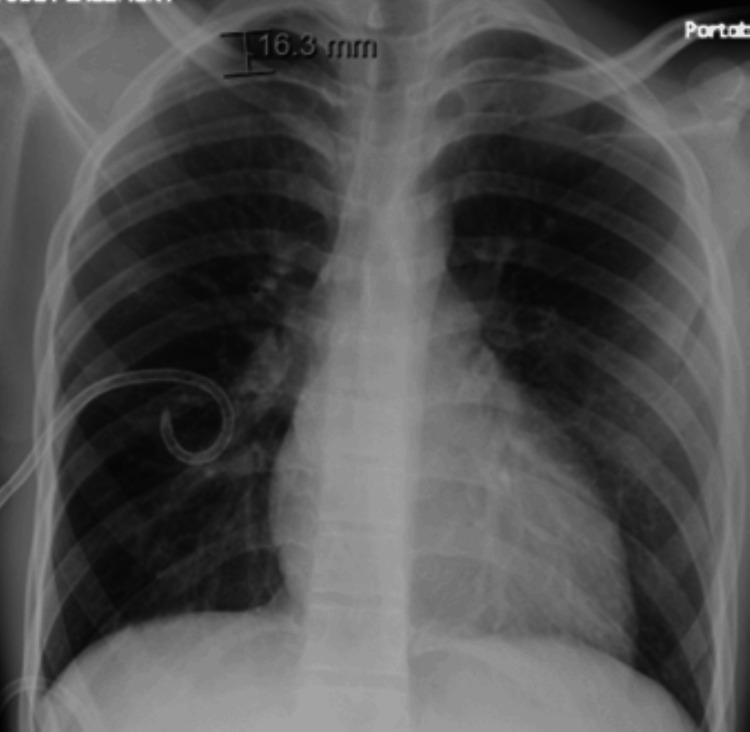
Chest radiograph following pigtail catheter placement confirming catheter position, lung re-expansion, and resolution of the PTX Note the reduction in PTX compared to Figure 1. PTX: pneumothorax
